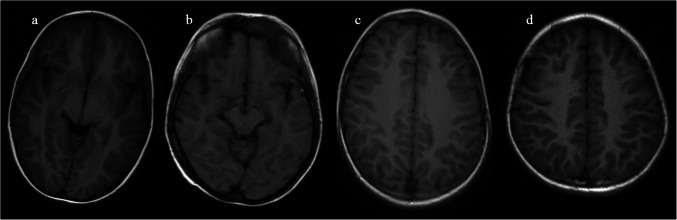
Axial unenhanced T1-weighted magnetic resonance images from control (a, b) and intervention (c, d) patients rated as 2 in an 8-year-old girl (a), 3 in an 8-year-old boy (b), 4 in a 7-year-old girl (c), and 5 in an 8-year-old boy (d) on a 5-point scale, where higher scores reflect better image quality
